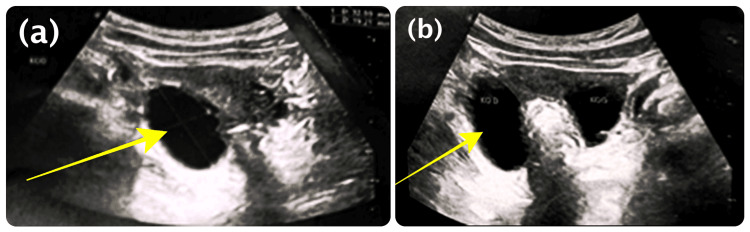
Pelvic ultrasound demonstrating ovarian cysts (a) Well-defined anechoic ovarian cyst (arrow), consistent with a functional cyst. (b) Bilateral ovarian cysts of varying sizes.
